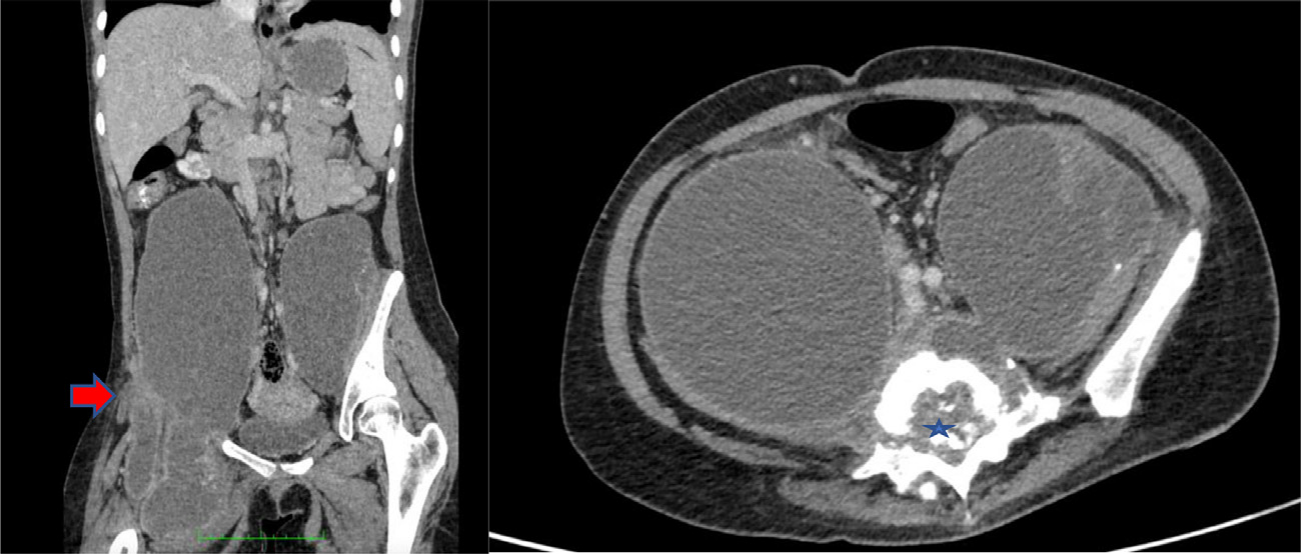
Contrast-enhanced abdominopelvic CT: (A) coronal plane demonstrated an extensive abscess in prevertebral space and revealed a psoas abscess in the right-left up to right inguinal fossa (red arrow); (B) the axial plane showed a retro vertebral abscess suggesting compression of the thecal sac (blue star)
